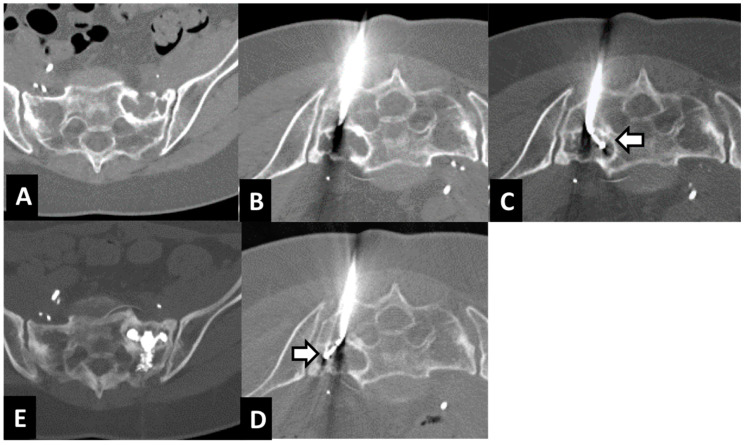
Treatment with tRFA and osteoplasty of a sacral metastasis from breast cancer in a 62-year-old woman. Axial CT scan showed a 37 mm lytic metastasis of the left sacral ala (A). The metastasis was reached with a 10-gauge Stabilit cannula (B). Axial CT showed two different placements of the steerable radiofrequency bipolar device (tSTAR) covering the entire lesion (arrows) (C,D). Axial CT scan showed the metastasis filled by PMMA (E).
